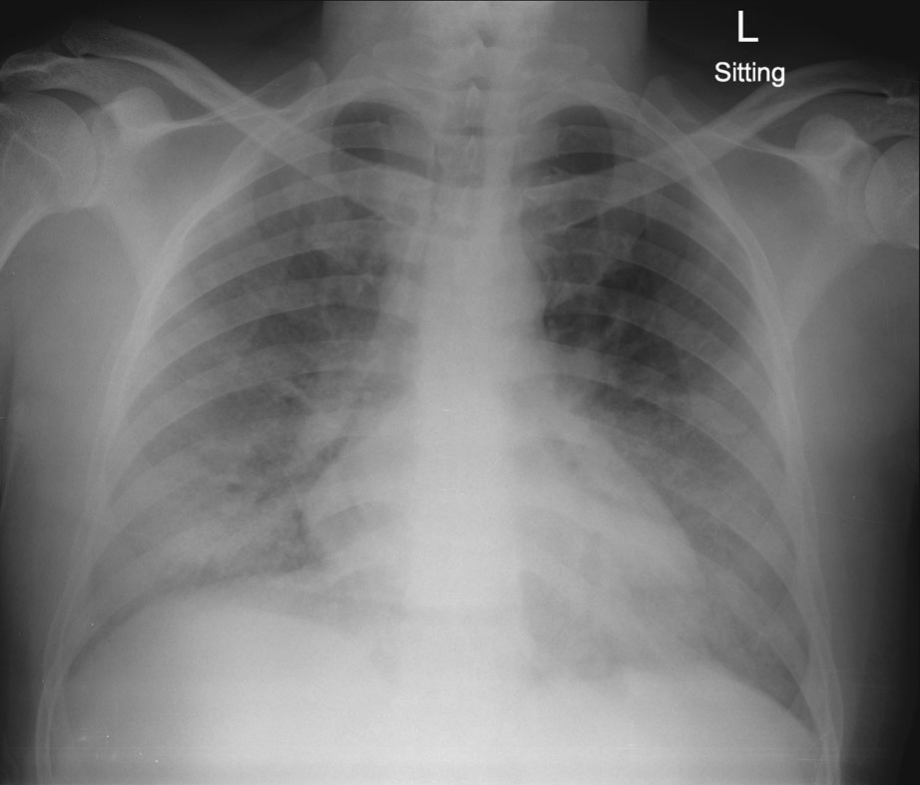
The patient's chest radiograph on admission showed bilateral, predominantly lower zone, ground‐glass opacifications.
